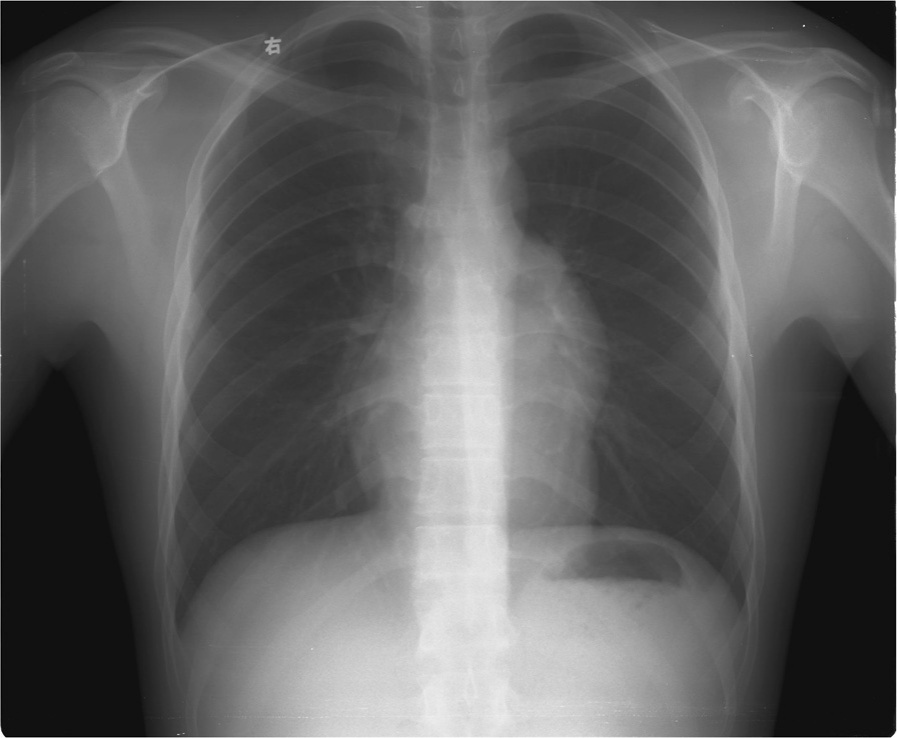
Imaging. Chest X-ray scan reveals a limited semicircle shadow located in the left heart edge.
